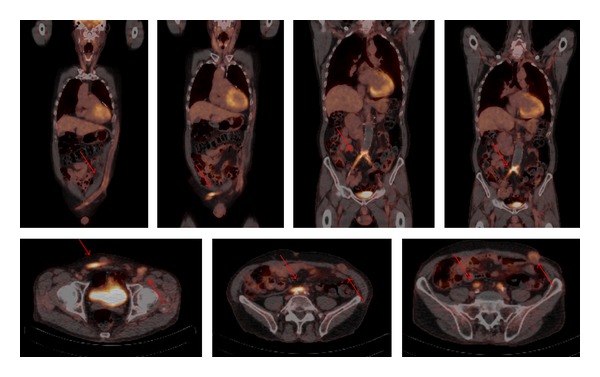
Coronal and axial view of fused ^18^F-FDG PET/CT images. In this particular case, a 67-year-old male patient underwent an aorto-bi-iliac bypass 16 years ago. After 3 years the bypass was revised because of an occlusion. Five years later the second bypass also occluded and an axillobifemoral bypass was constructed. Unfortunately this bypass also occluded twice. Patient is admitted to the hospital because of pain and redness at the level of the axillobifemoral bypass probably due to infection. ^18^F-FDG PET/CT scanning showed increased FDG uptake at the level of both occluded bypasses, the aorto-bi-iliac bypass and the axillobifemoral bypass. Arrows are pointing at increased FDG uptake in both bypasses. Ultimately, no bacteria were cultured.
